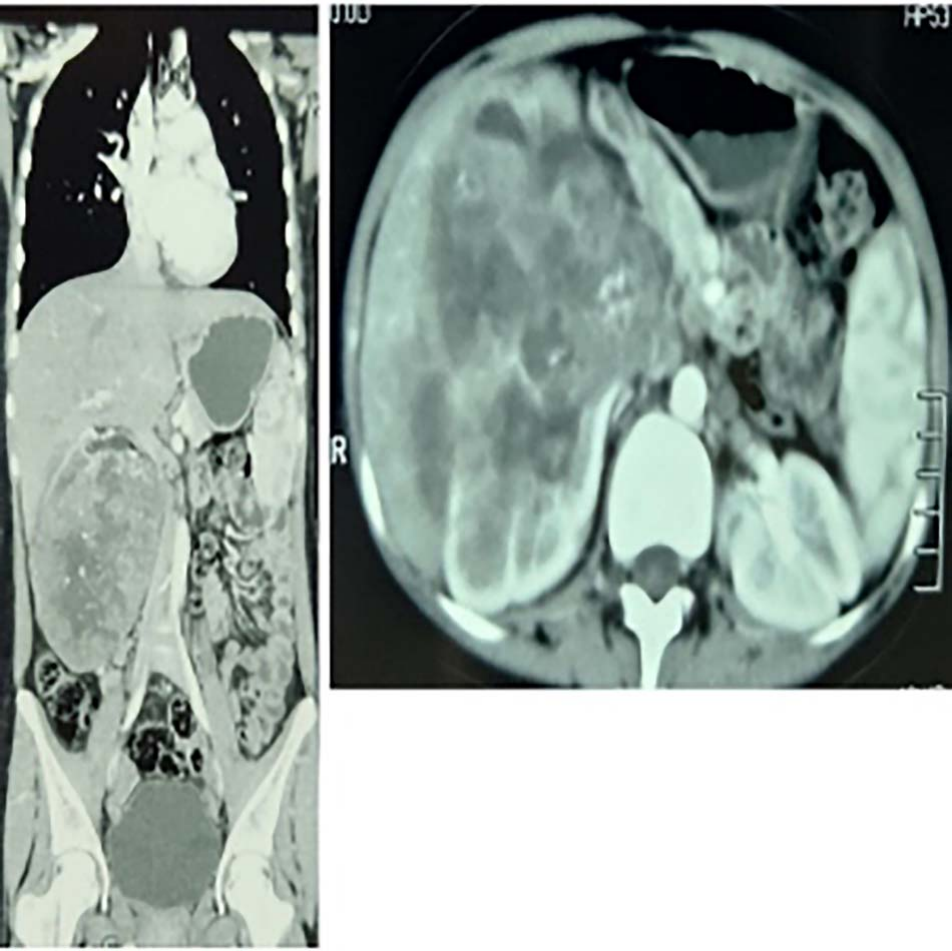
To the left: coronal section multiple-slice computed tomography slice showing the lump at the greatest diameter, to the right a transverse section showing a renal mass.
